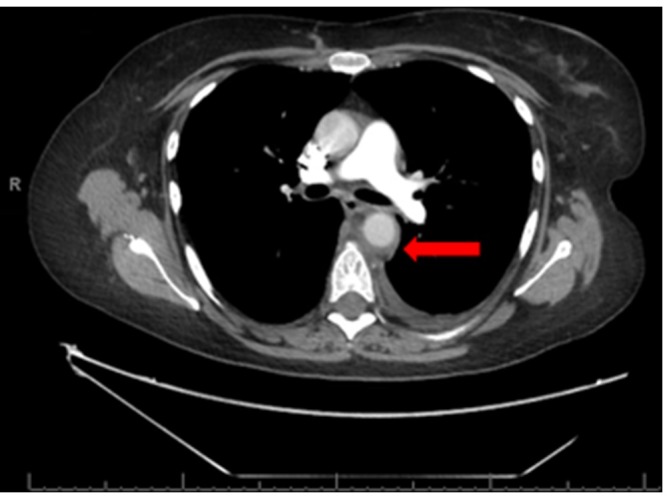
Axial CT with i.v. contrast. Abnormal circumferential mural thickening is
